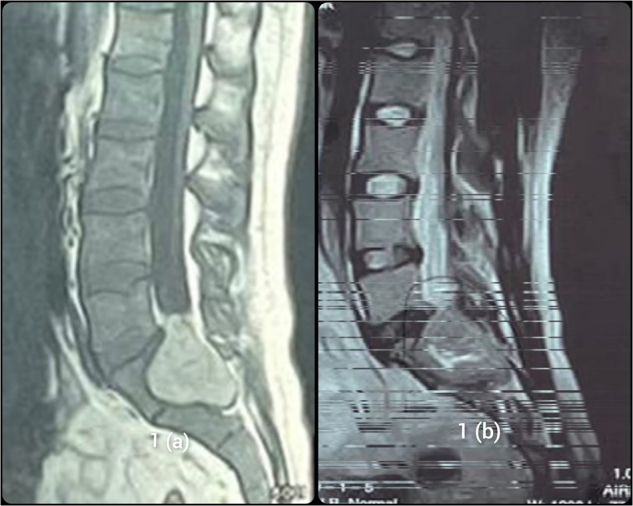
(a) and (b) show the sagittal sections of T1- and T2-weighted images, respectively.
